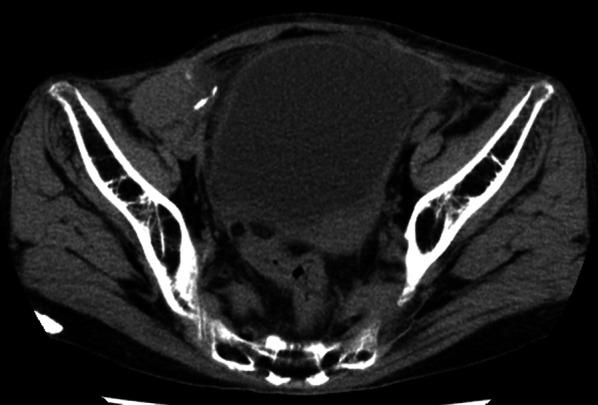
Abdominal computed tomography. A tense bladder is revealed on computed tomography
